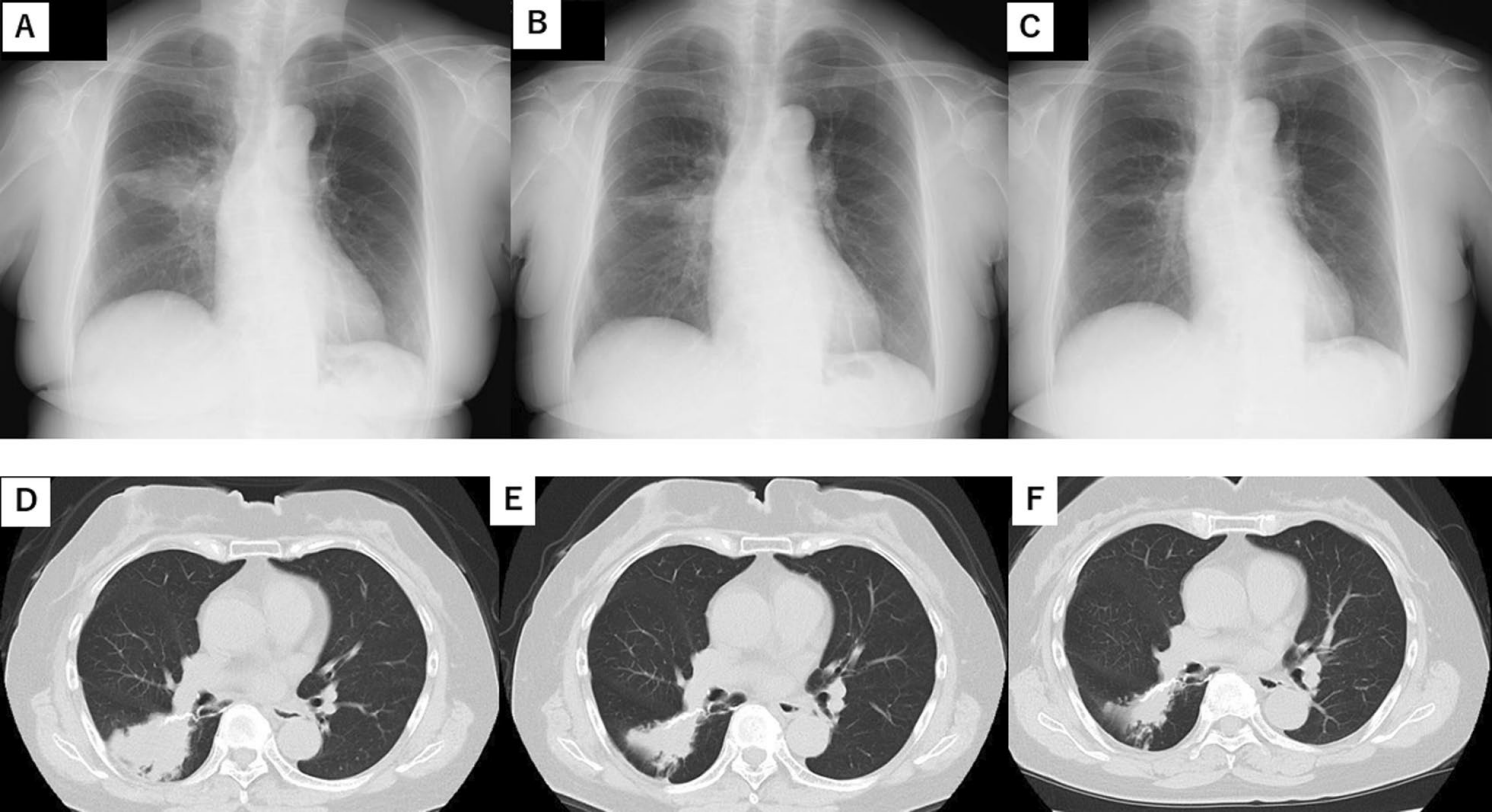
Radiographic findings for case 2. A, D Chest radiograph and computed tomography (CT) image six months after partial resection of the right lower lobe shows the shadow of a suspected mass. B, E Imaging shows that the shadow shrunk four months after the use of low-dose erythromycin. C, F Chest radiograph and CT image show well-controlled inflammatory change after one year
